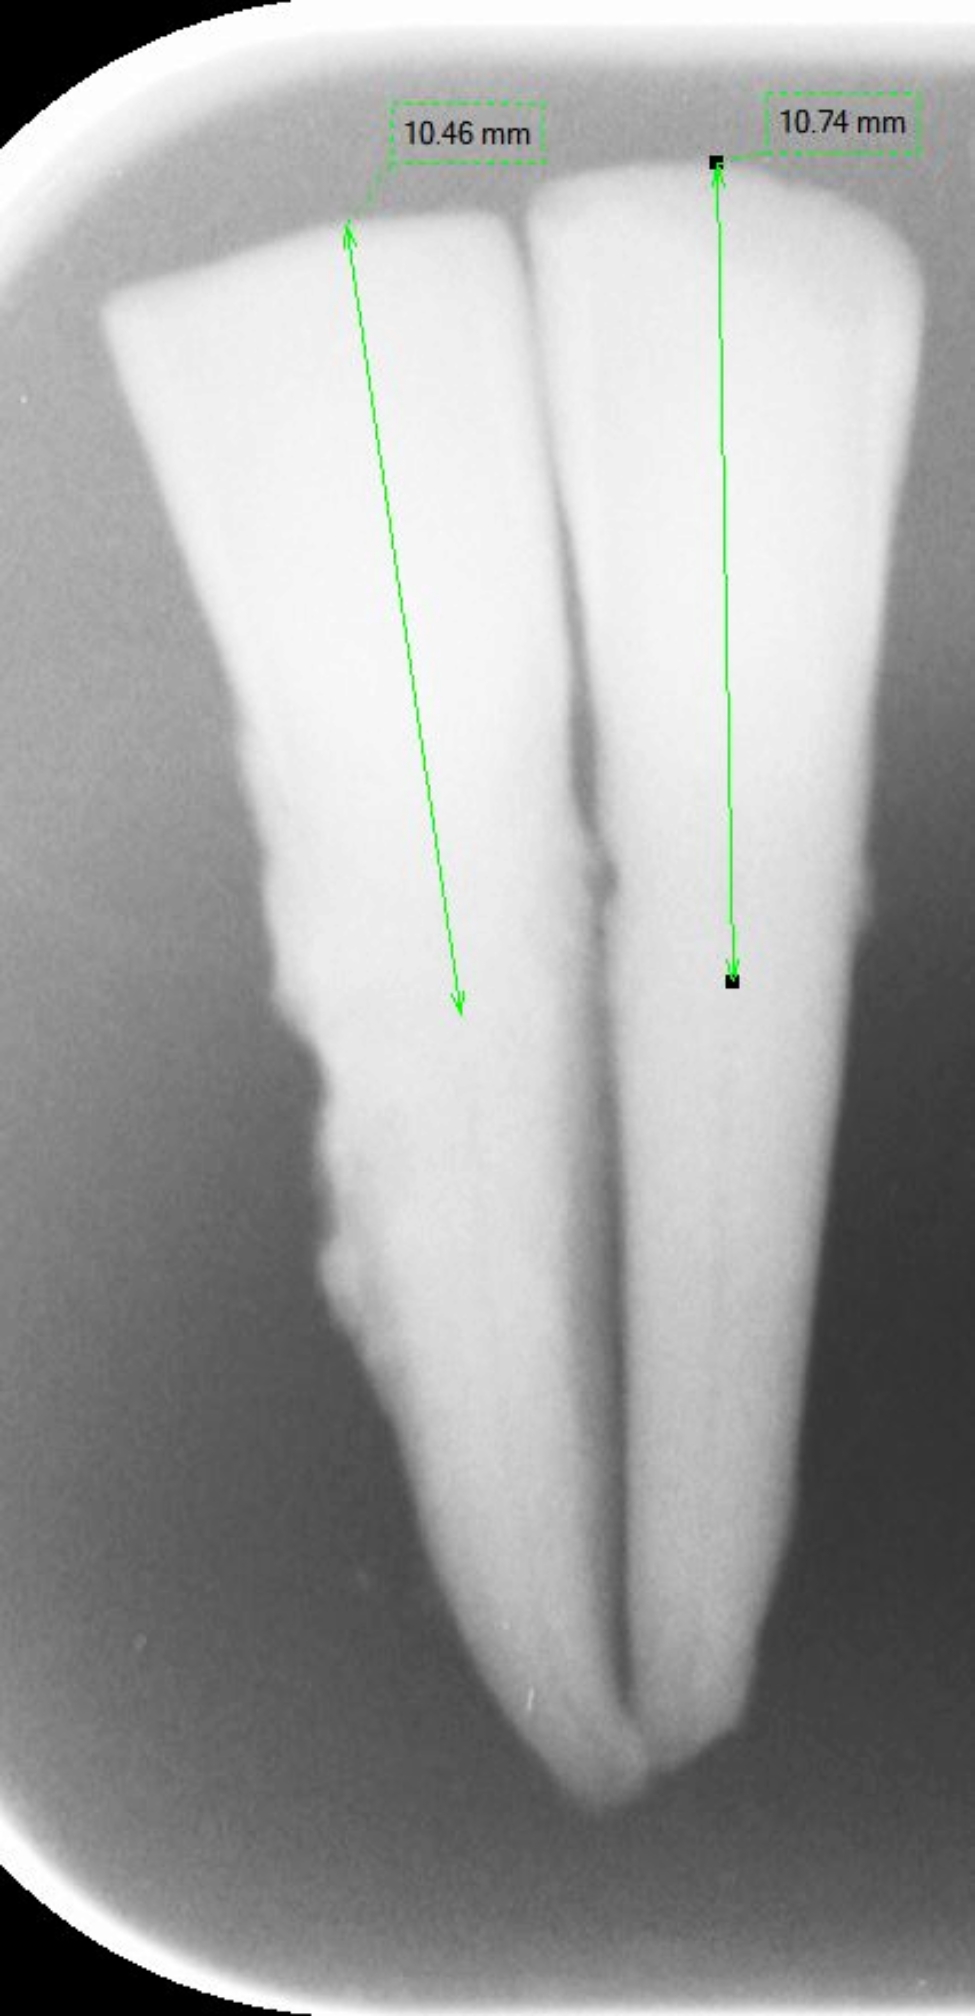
Two-dimensional X-ray of a matched pair of teeth. The incisal-pulpal distance of matched teeth could not differ by more than 10%
